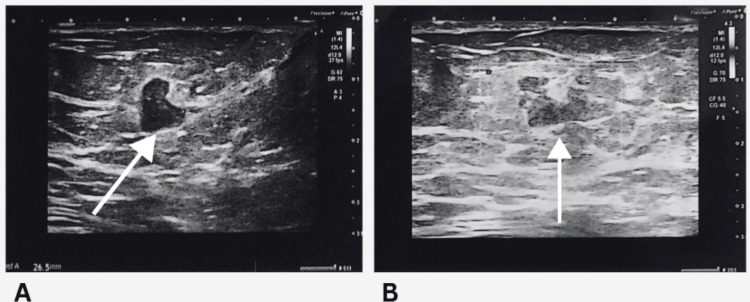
Ultrasound images showing a hypoechoic lesion within a dilated duct, suggestive of intraductal papilloma versus low-grade DCIS. (A) Longitudinal view revealing a well-defined, lobulated hypoechoic lesion (arrow) within a markedly dilated duct. (B) Transverse view showing the same hypoechoic lesion (arrow) embedded in heterogeneous surrounding parenchyma, consistent with ductal involvement. DCIS: ductal carcinoma in situ.
